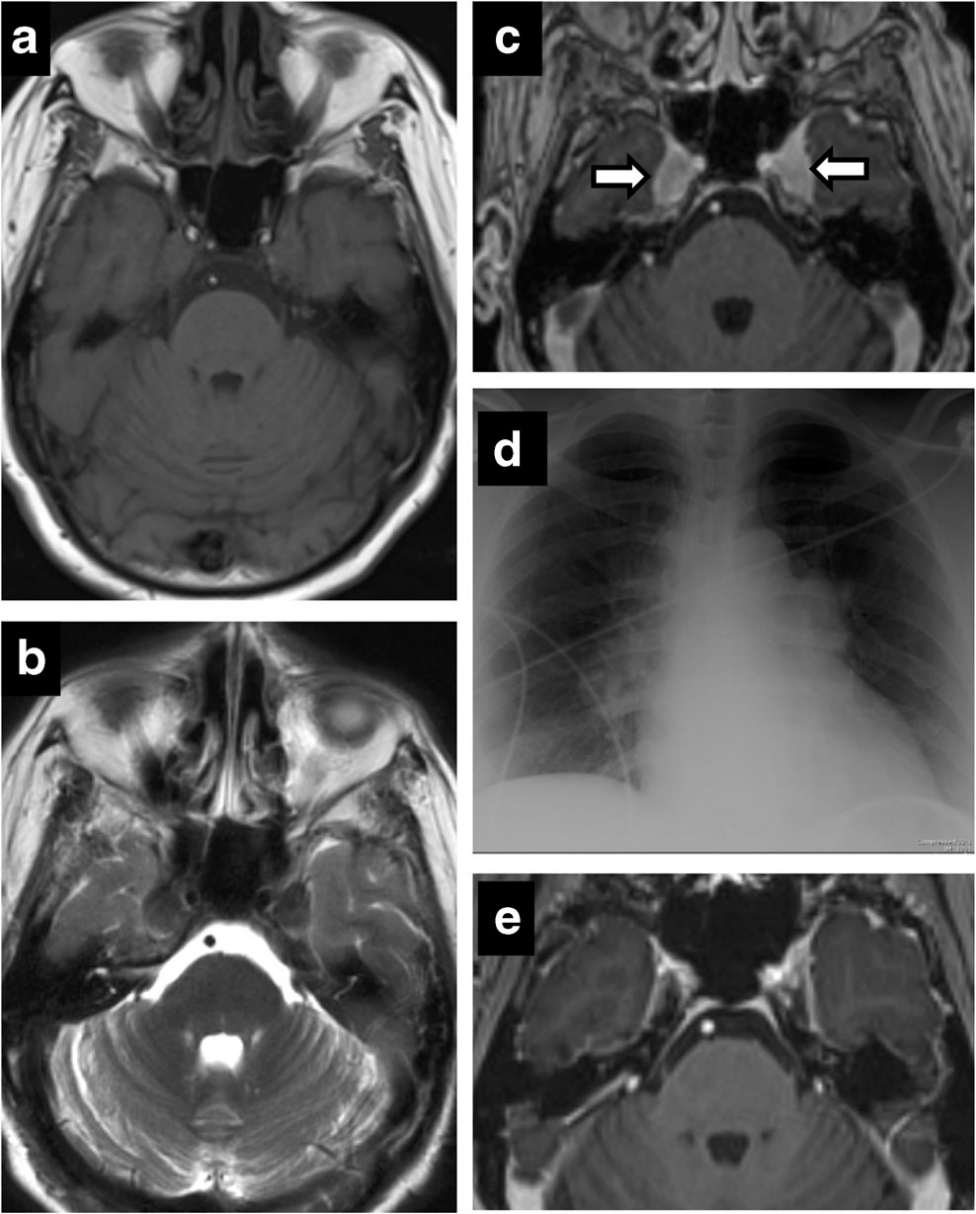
Sarcoidosis. Axial T1 (a), axial T2 (b), axial T1 C+ (c). A 53-year-old woman with facial pain and numbness. Enhancing, T2 hypointense lesions symmetrically involving the bilateral Meckel’s cave. Chest X-ray (d)—bilateral hilar and mediastinal lymphadenopathy. Follow-up (e)—complete resolution
